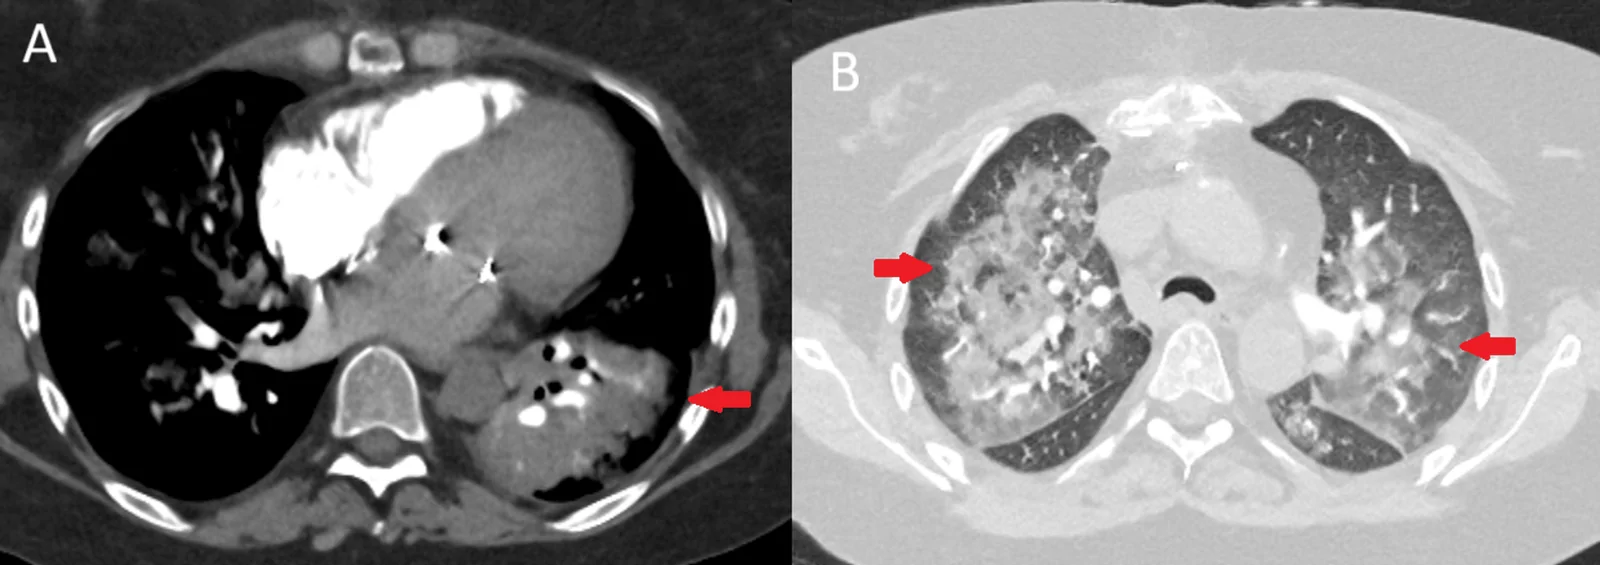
CT pulmonary angiography. (A) Mediastinal window demonstrating a large dense consolidation in the left lower lobe (arrow), with additional patchy opacities in right lung. (B) Lung window confirming extensive left lower lobe consolidation with extensive bilateral ground-glass opacities (arrows), consistent with severe hospital-acquired pneumonia. No pulmonary embolus was identified.
